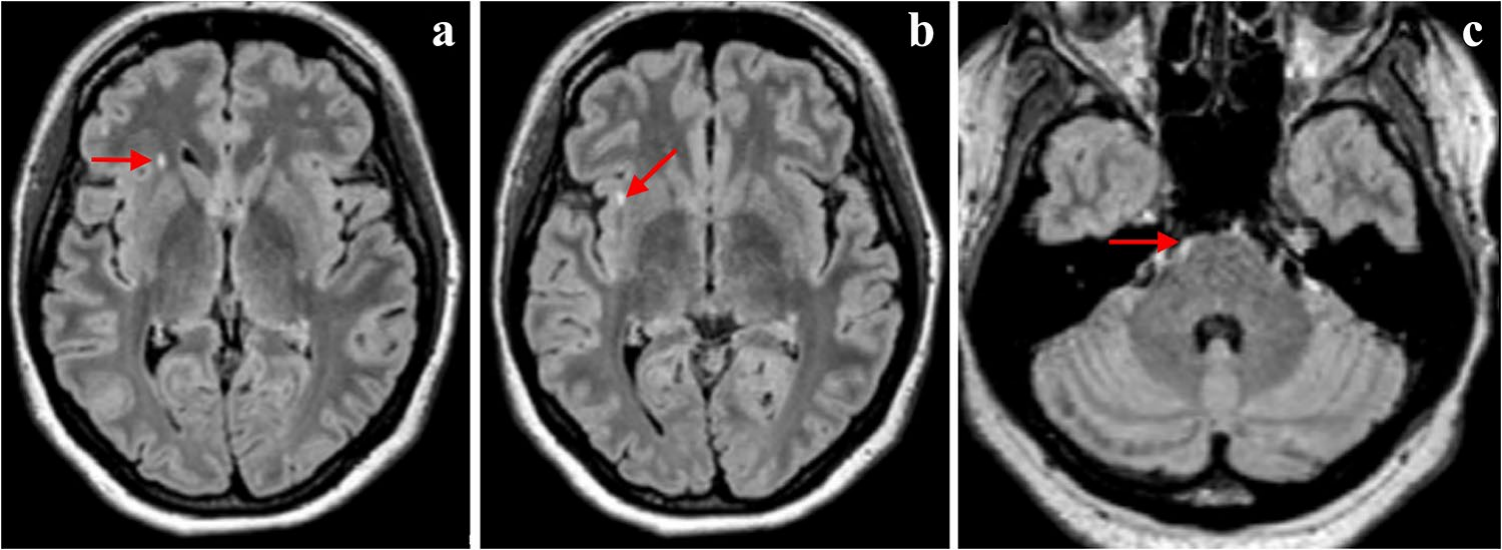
MRI of the patient 3.0. Discrete pathological MRI involvements fulfil MRI criteria with typical lesions distribution for MS. Axial FLAIR show small lesions in the frontal lobe (a, b), one in the typical subcortical region (b) and in the pons (c)
